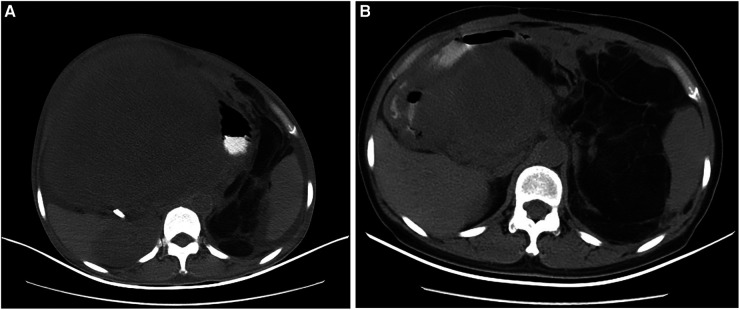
The imaging of CT scanning indicated for the second and third recurrence of the female patient in July 2010 and November 2011, respectively. (A) The giant tumors with adipose density occupied almost the entire abdominal cavity (July 2010); (B) The tumors originated from the hepatic hilar region and oppressed adjacent organs and tissues (November 2011).
